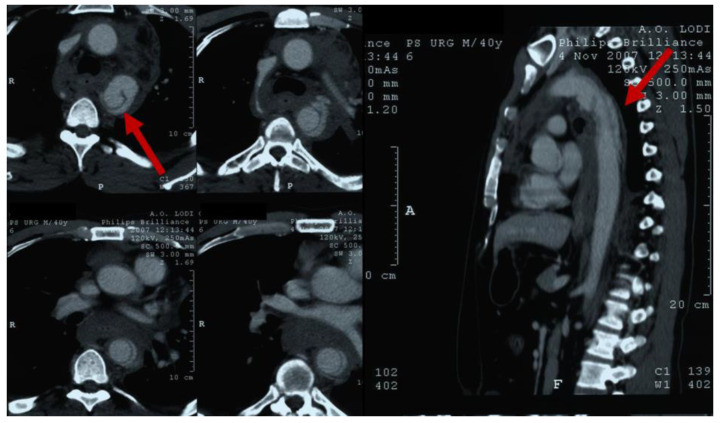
Angio-Computed Tomography chest scan of a patient with BTAI at isthmus, causing intimal tear (red arrow) in axial (on the left) and sagittal (on the right) views.
